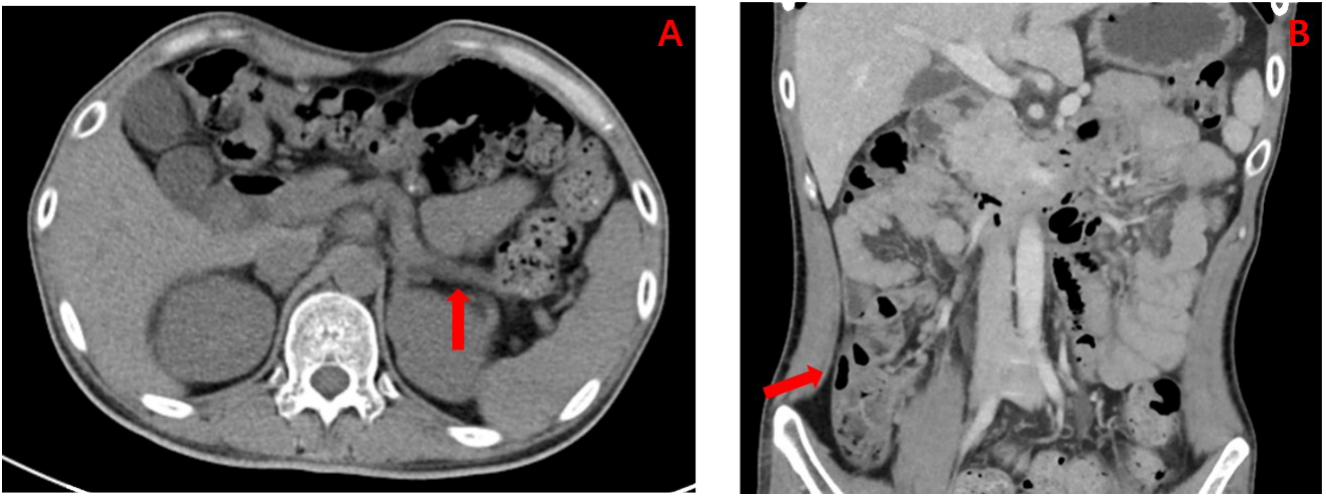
(A) Enhanced CT abdomen showing the result of dorsal pancreatic agenesis (as indicated by the red arrows; considering pancreatic developmental variation), an exceedingly rare congenital disease reported in the literature. (B) Enhanced CT abdomen showing the result of multiple complex renal cysts (as indicated by the red arrows).
